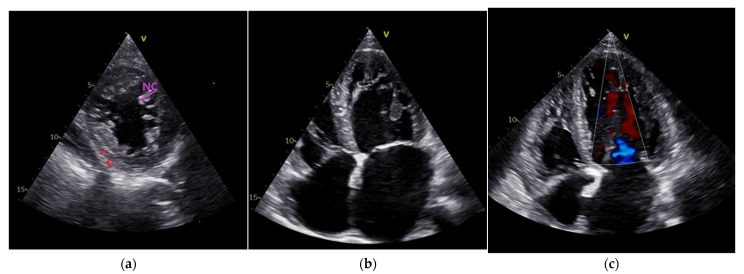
(a) Transthoracic echocardiography. Parasternal short-axis view. Non-compacted–NC/Compacted-C ratio > 2; (b) Apical 4 chamber view. Trabeculations at the apex and lateral wall of the LV, dilated left atrium. (c) Transthoracic echocardiography. Color flow in the intertrabecular recesses.
